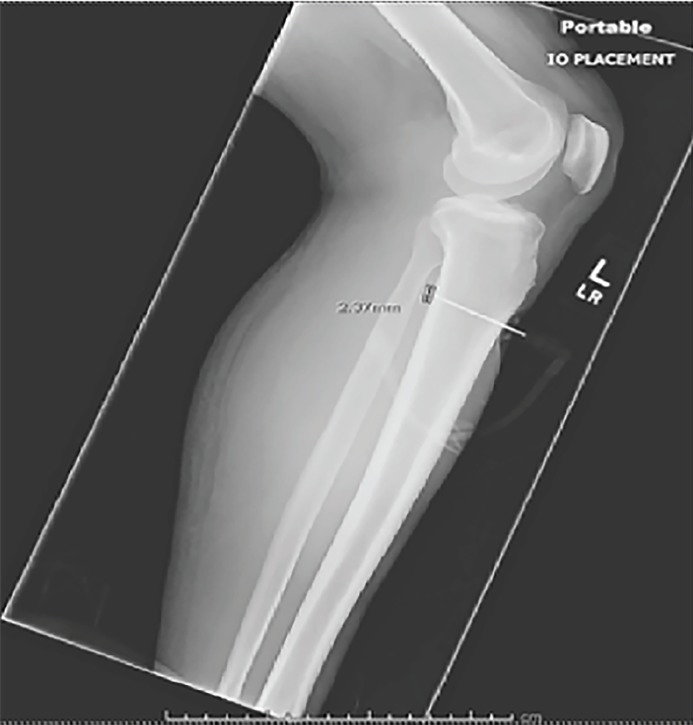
Radiographic demonstrating intraosseous needle extending 2 mm past posterior cortex of tibia.
